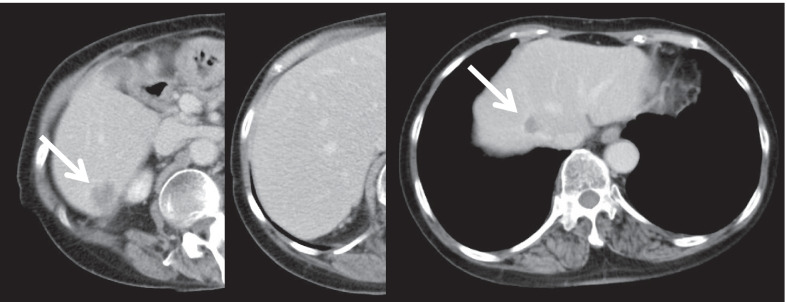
Abdominal CT scan showing reduction of liver metastases by chemotherapy (white arrow). *CT* computed tomography
